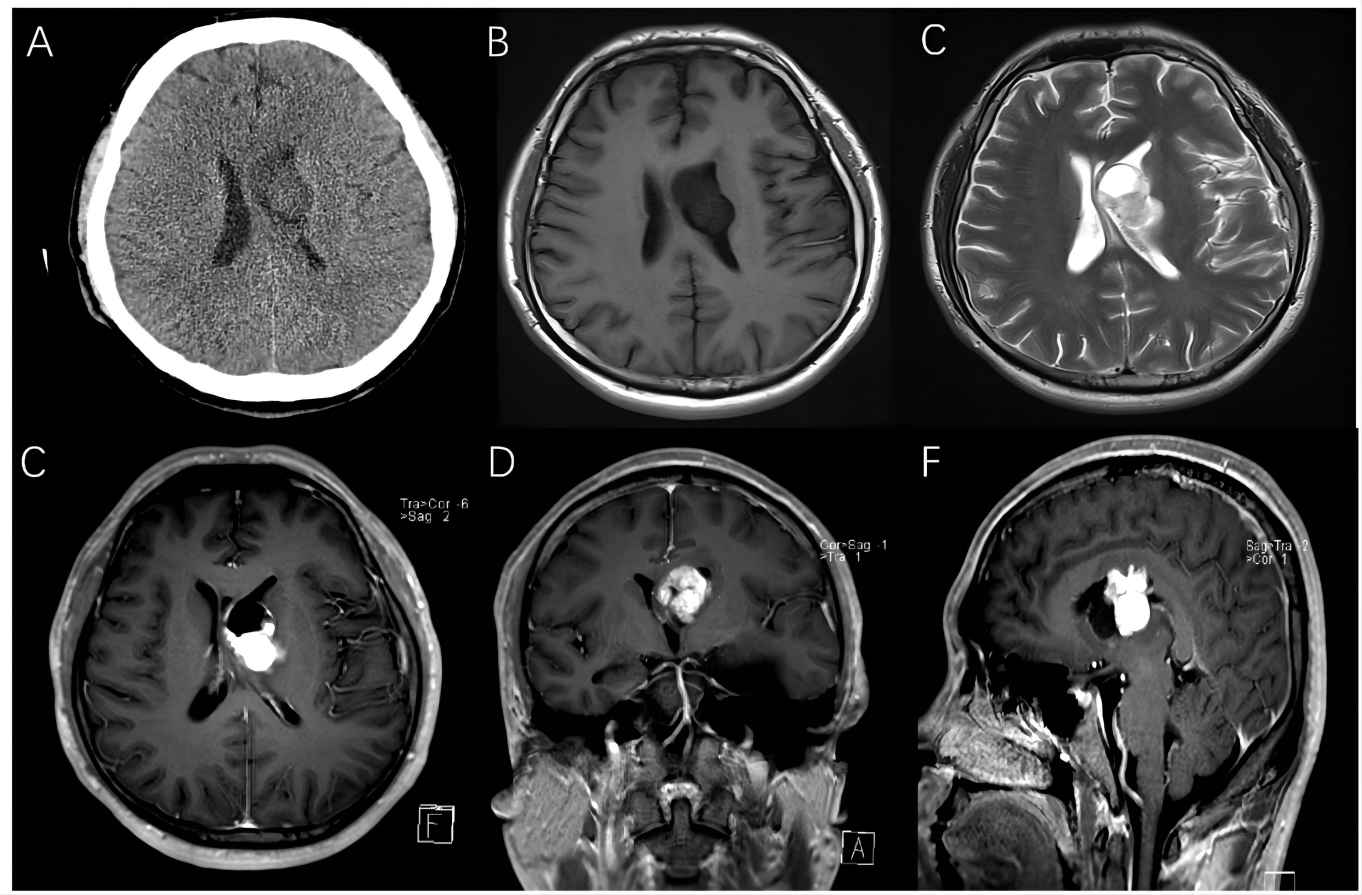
Preoperative images of a 27-year-old man with intraventricular hemangioblastoma. (A) CT scans showed a 3.5 cm hypo-isodense mass located in the left lateral ventricle. (B–F) MRI revealed a solid-cystic mass, and the solid part of mass was mildly heterogeneously enhancing.
